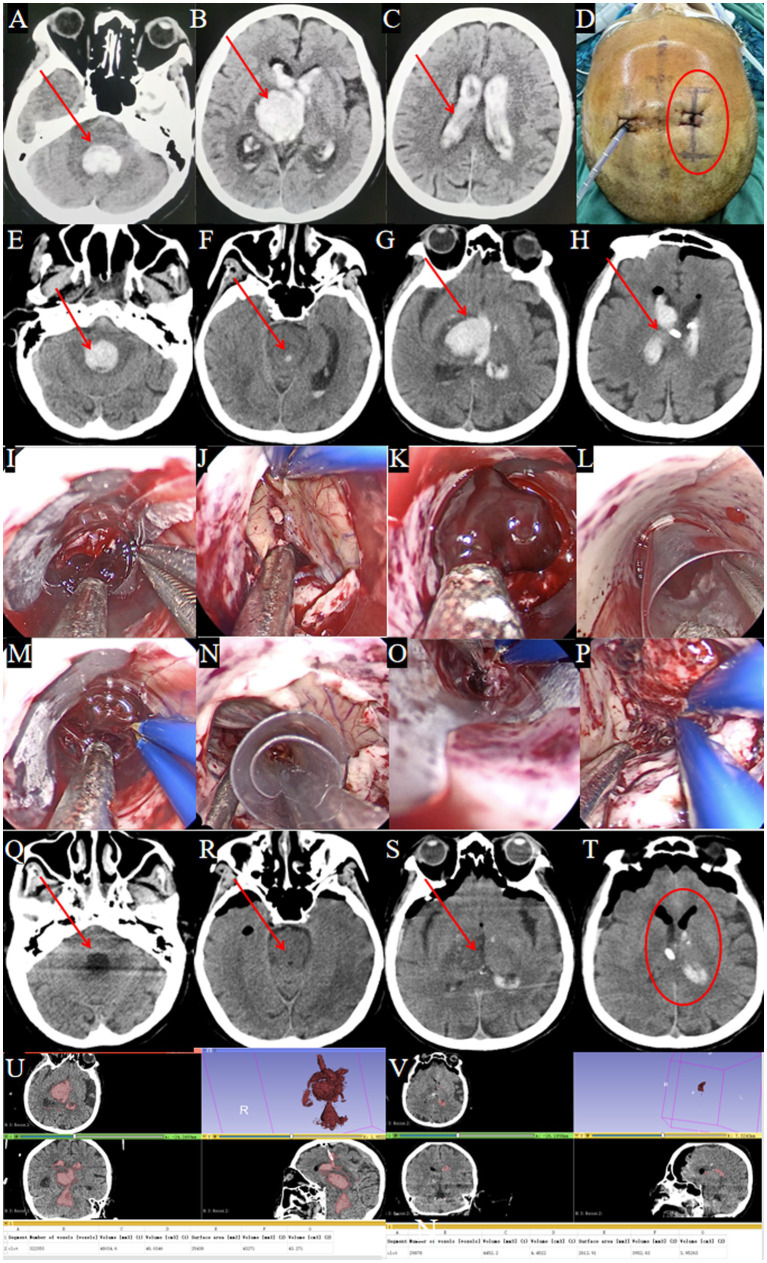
Surgical procedure using the TSNCT for complete ventricular casting. (A–D) Patient transferred after EVD at a local hospital (→ arrows in A–C show residual clots in fourth, third and lateral ventricles; ◯ circles in D mark the right frontal horn approach site). (E–H) Admission CT showing complete ventricular casting (→ arrows indicate hemorrhage in all ventricles). (I) Insertion of the outer sheath through the puncture pathway. (J) Hematoma removal from the lateral ventricle. (K) Clearance of hematoma at the foramen of Monro. (L) Insertion of the middle sheath into the third ventricle. (M) Exploration of the midbrain aqueduct. (N) Insertion of the mini-sheath through the midbrain aqueduct. (O) Hematoma removal from the fourth ventricle. (P) Endoscopic view confirming complete evacuation of the fourth ventricle. (Q–T) Postoperative CT showing successful hematoma clearance (→ arrows denote evacuated ventricles; ◯ circles confirm restored anatomy). (U) Preoperative CT: hematoma volume = 48 mL. (V) Postoperative CT: hematoma volume = 4.5 mL.
